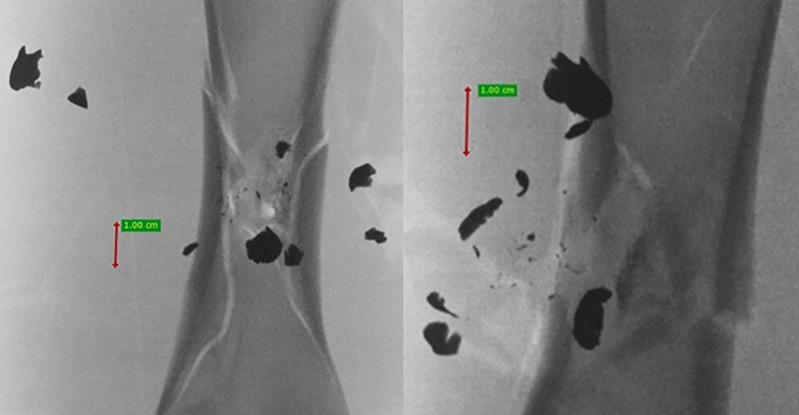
H&N Baracuda airgun pellet cal. 6.35 mm, on the left: A-P X-ray, on the right: lateral X-ray. Shot to the posterior surface of the femoral shaft.
